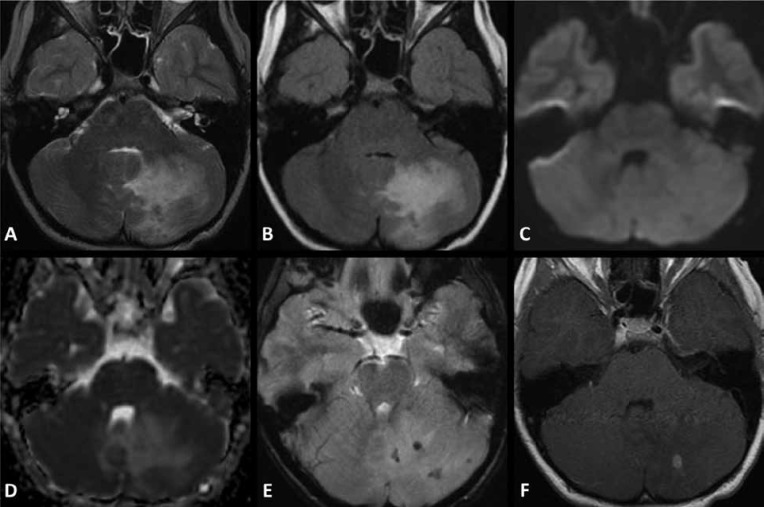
Third MRI at age eight. (A) Axial T2- weighted image, (B) axial FLAIR image showing continued increase in size of the patchy areas of T2 prolongation in the left cerebellar hemisphere, (C) axial diffusion weighted image, (D) axial ADC map show corresponding increased diffusivity of the parenchymal lesions, (E) small foci of increased magnetic susceptibility on GRE T2* images are present and (F) post contrast axial T1-weighted image showing persistent leptomeningeal enhancement with more confluent nodular parenchymal enhancement.
